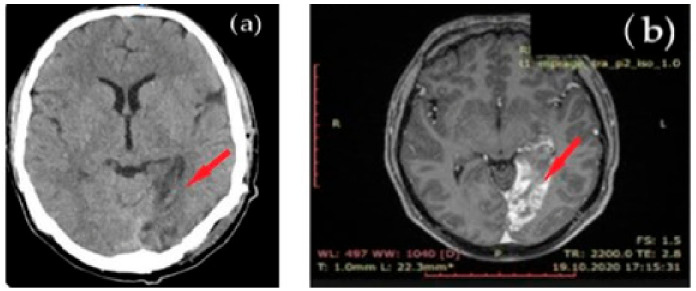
(a,b): Cerebral imaging. (a) Native brain CT aspect: left occipital ischemic hypodense lesion; (b) brain MRI aspect: left occipital ischemia.
